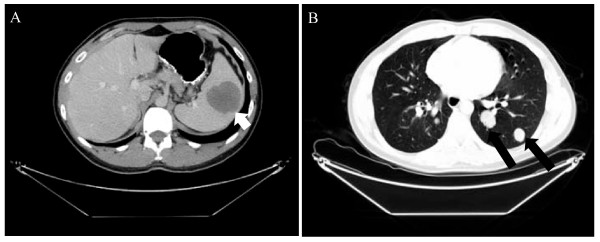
Computed tomography revealed splenic lesion (A, identified with arrow) and demonstrating metastatic lung lesions (B, identified with arrows).
